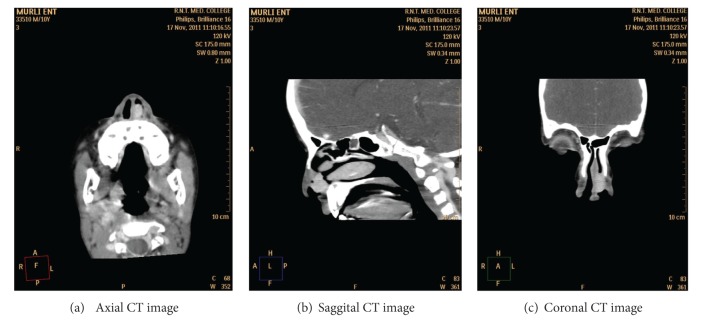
Axial, sagittal, and coronal CT images showing an intensely enhancing homogenous mass lesion in the left vestibule.
